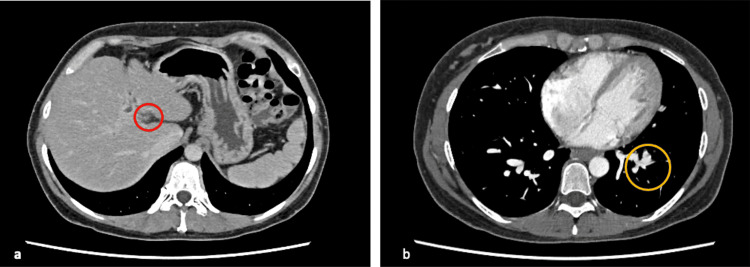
Contrast-enhanced CT performed at 17 weeks and 14 days a: Extrahepatic portal vein occlusion (red circle); b: Left pulmonary venous fistula (yellow circle)
